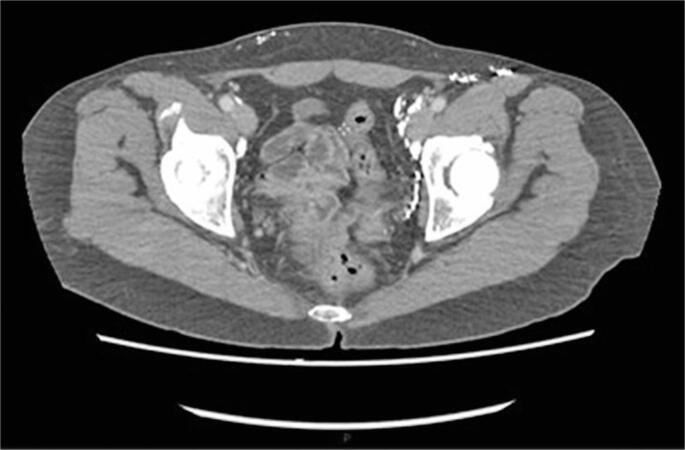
Axial view of control computed tomography image generated approximately six months after transafferent nodal embolization (TNE). The image shows the absence of free fluid in the pelvis and the TNE-related radiopaque material next to the pelvic and inguinal retroperitoneal lymph nodes
